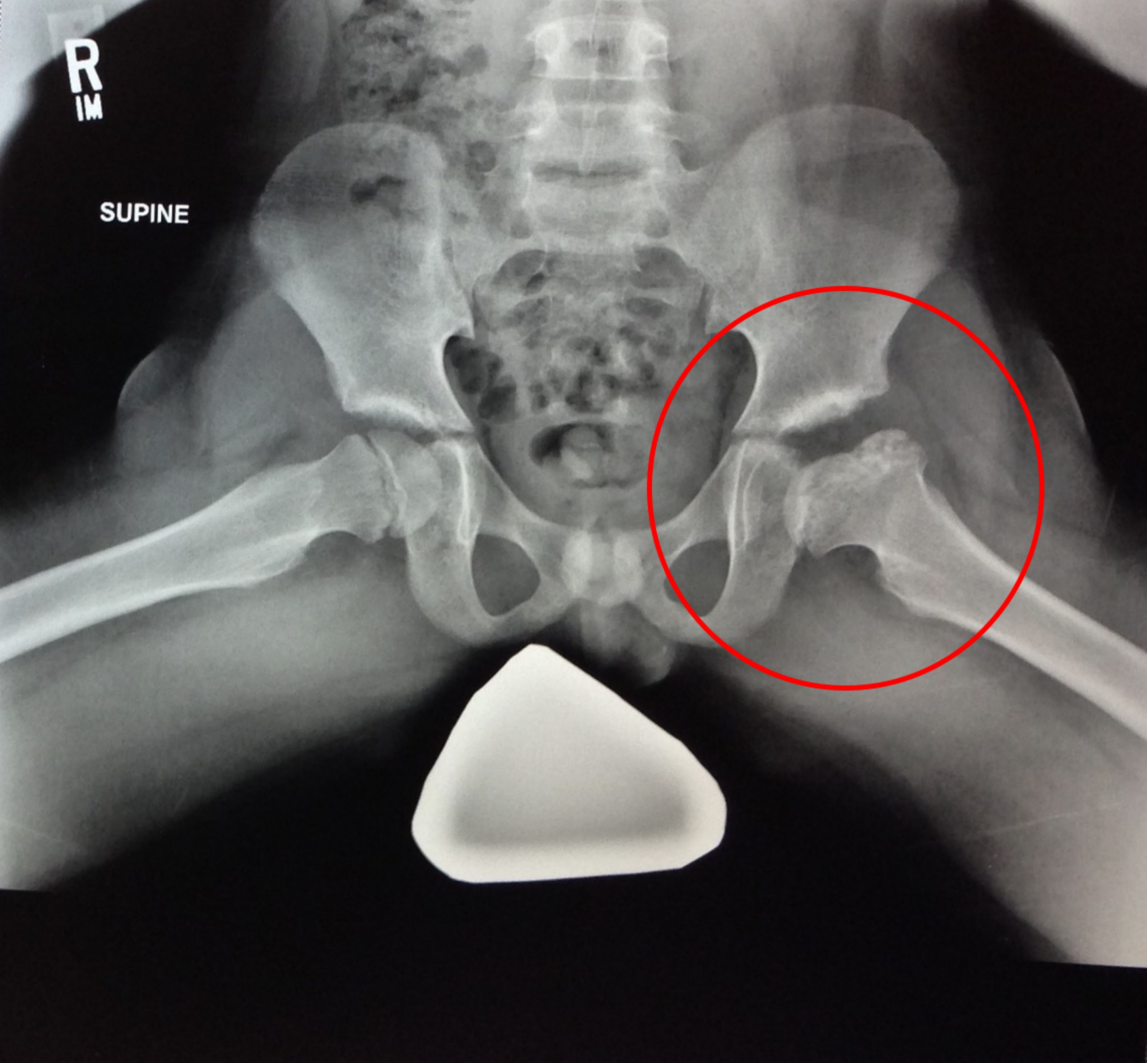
Frog leg lateral view pelvic X-ray taken on December 21, 2011.
